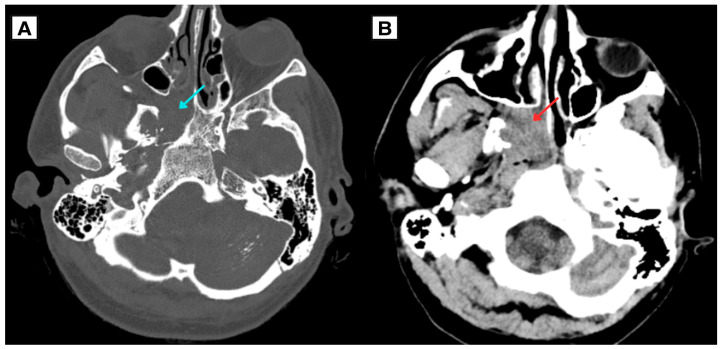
CT of the nasopharyngeal region. (A) Axial bone window demonstrates right-sided dilatation of the pterygoid (vidian) canal (blue arrow) and remodeling of the anterior wall of the sphenoid sinus. (B) Incidental suspicion of a right-sided nasopharyngeal mass (red arrow) with extension toward the pterygopalatine fossa.
